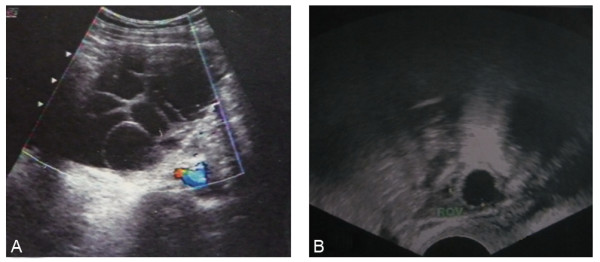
Pelvic ultrasound image showing right ovary before (A) and after thyroxine treatment (B).
